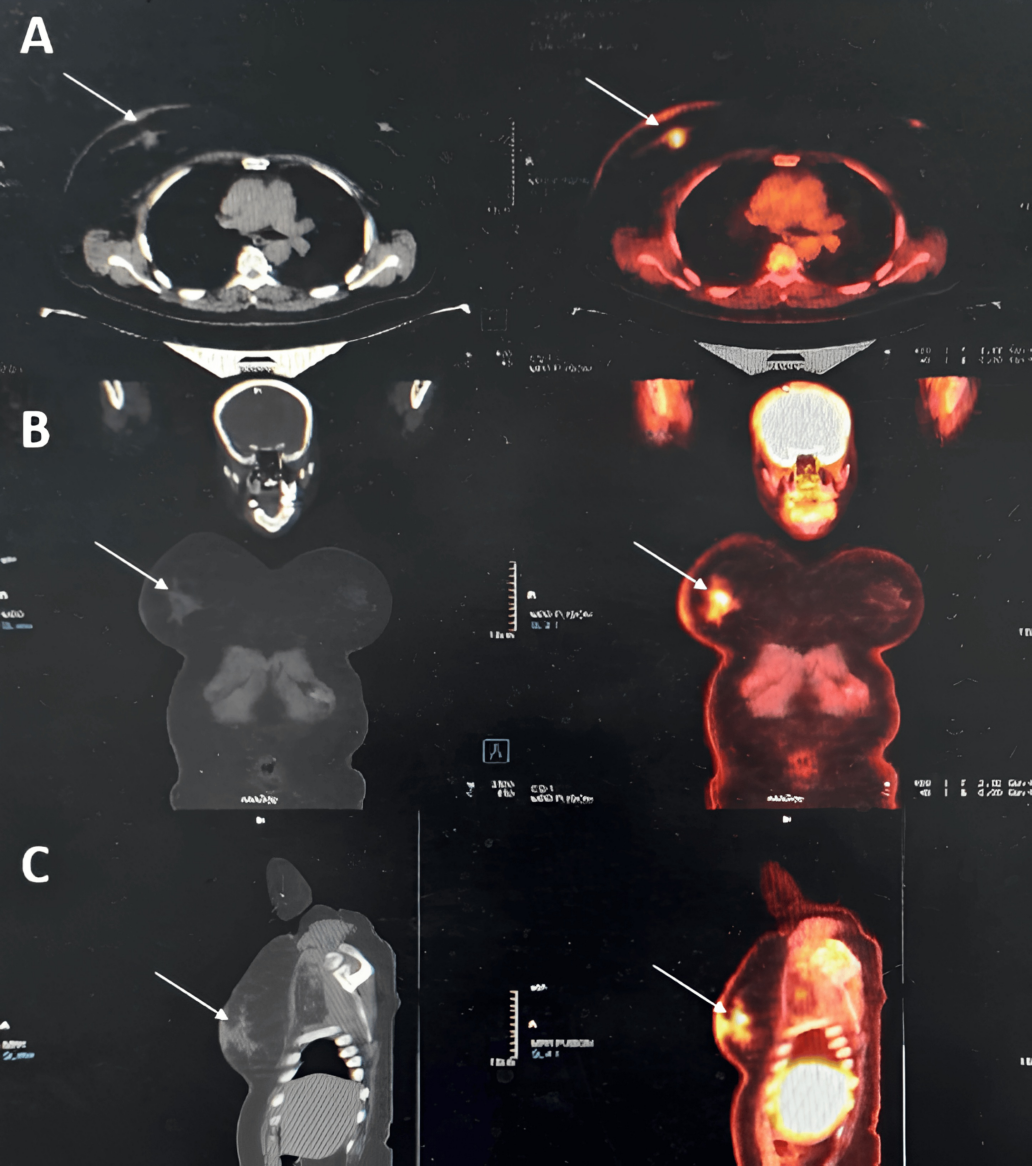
PET-CT images of the right breast Axial (A), coronal (B), and sagittal (C) sections show a hypermetabolic focus (white arrow) in the right breast located behind the nipple and corresponding to the known primary tumor.
